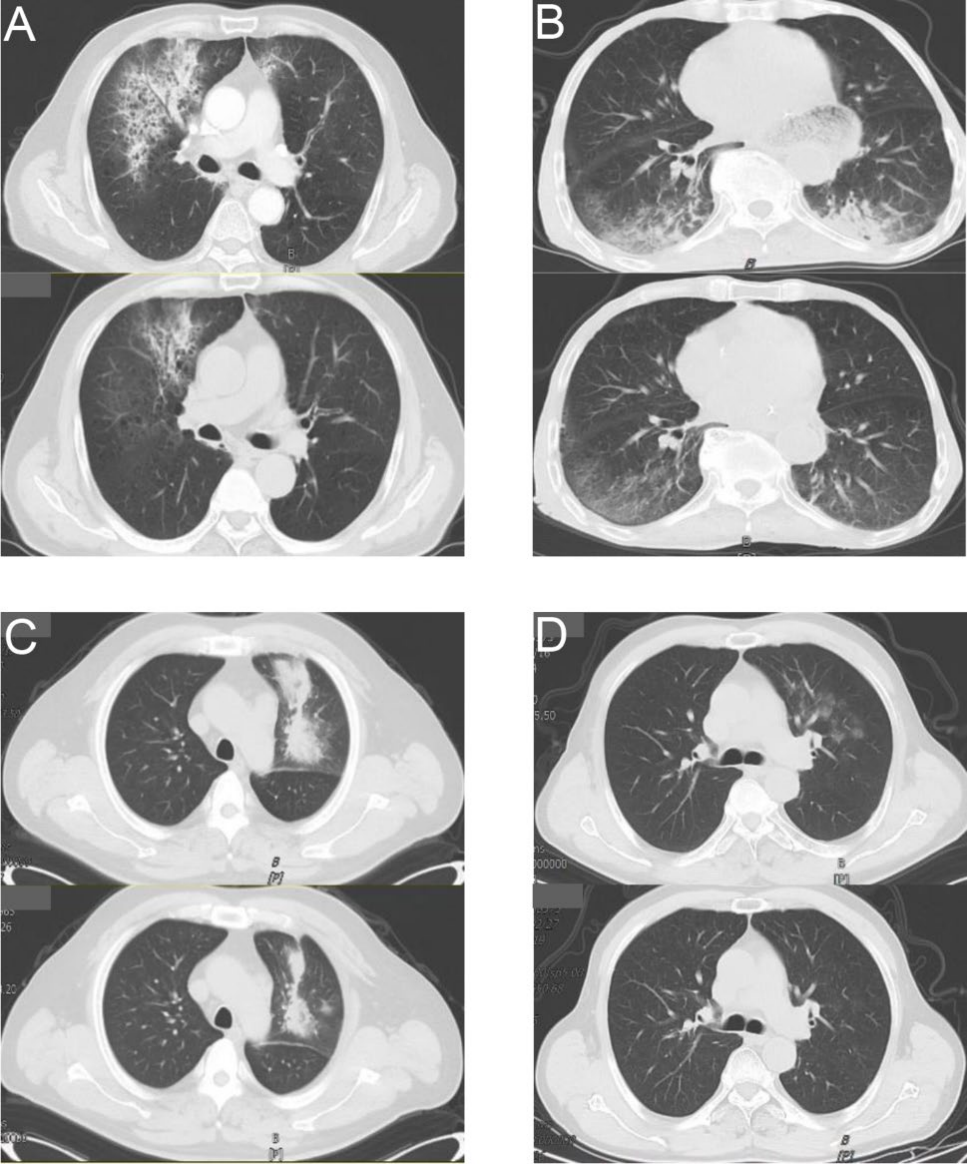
Optimal CT changes in several patients with COVID-19 pneumonia. Two CT slides of four cancer patients are shown respectively within 1-week EGCG treatment. The top is the baseline and the bottom is significantly absorbed and dissipated improved chest imaging
